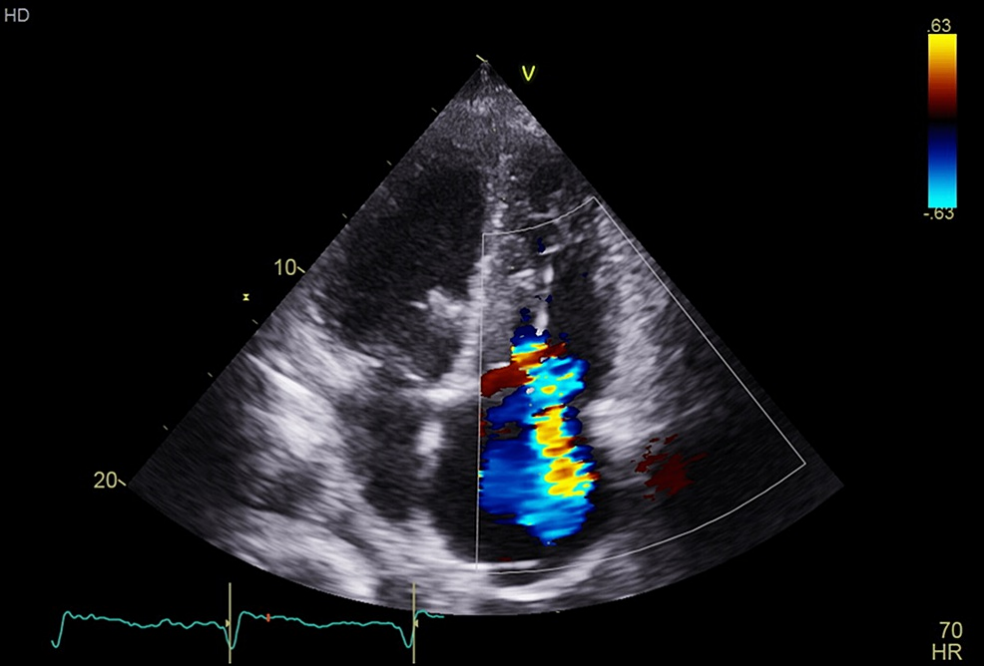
Repeat transthoracic echocardiogram at 10 months showing pacemaker lead and interval development of severe tricuspid regurgitation. By Mayo Clinic convention, the left ventricle is inverted on the image (left upper cardiac chamber on image).
